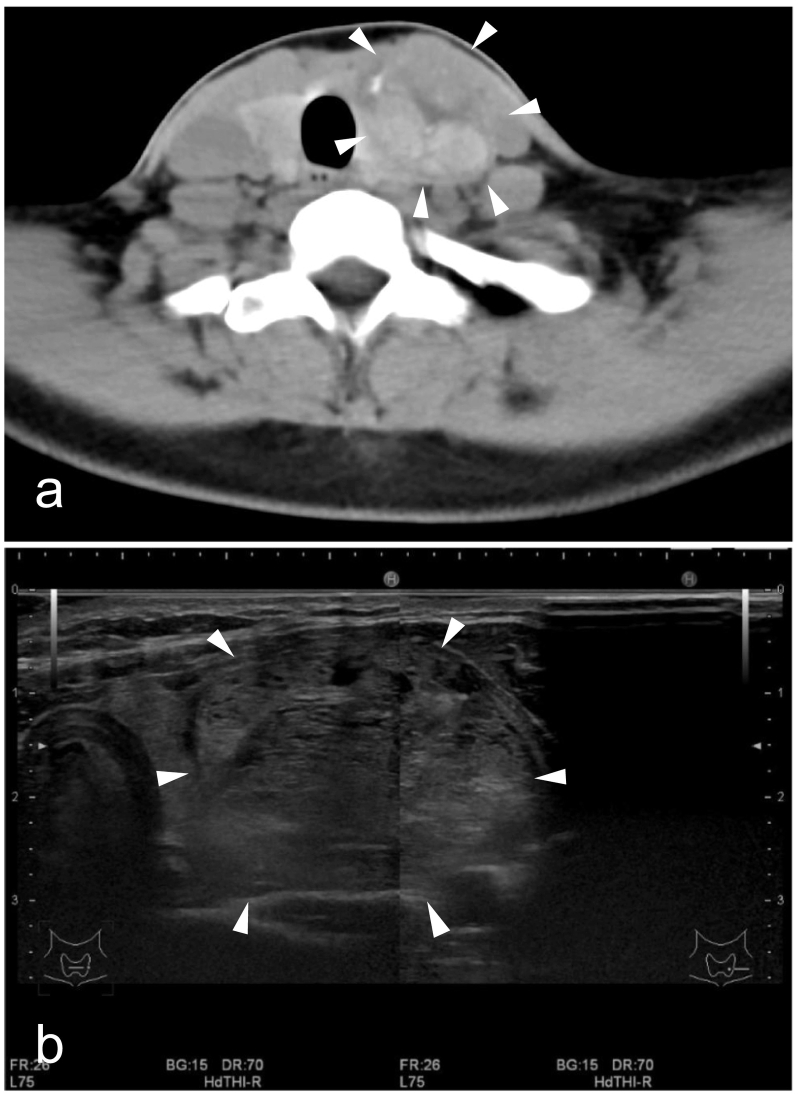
(a) Computed tomography image of a mass lesion (indicated by the arrowheads) with mixed low and high absorption profile in the left lobe of the thyroid gland. (b) Ultrasound image of the well-defined, smooth, and large tumor mass (indicated by the arrowheads) measuring 51 × 31 × 19 mm.
